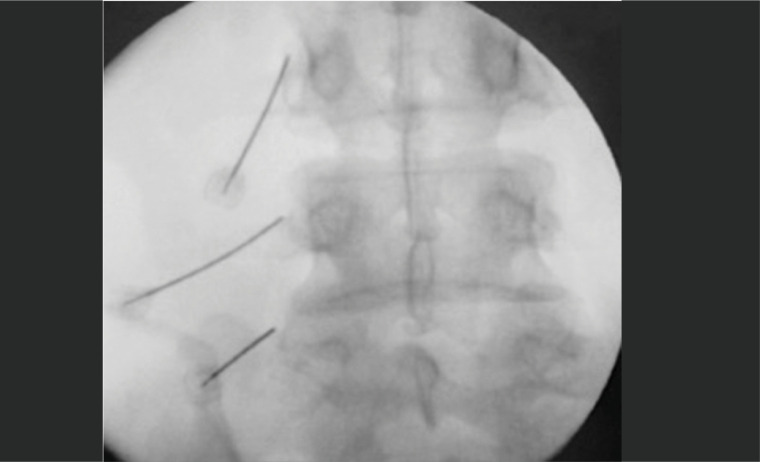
X-ray image of the procedure. X-ray image showing needle placement along the course of the medial branches of the dorsal rami at L3-L4, L4-L5, and L5-S1 on the left side.
